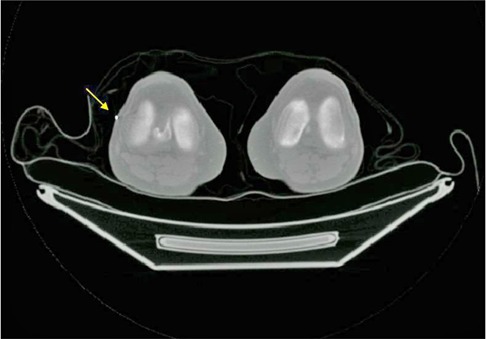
A typical CT image in transaxial FOV showing patient's two legs with a BB.
